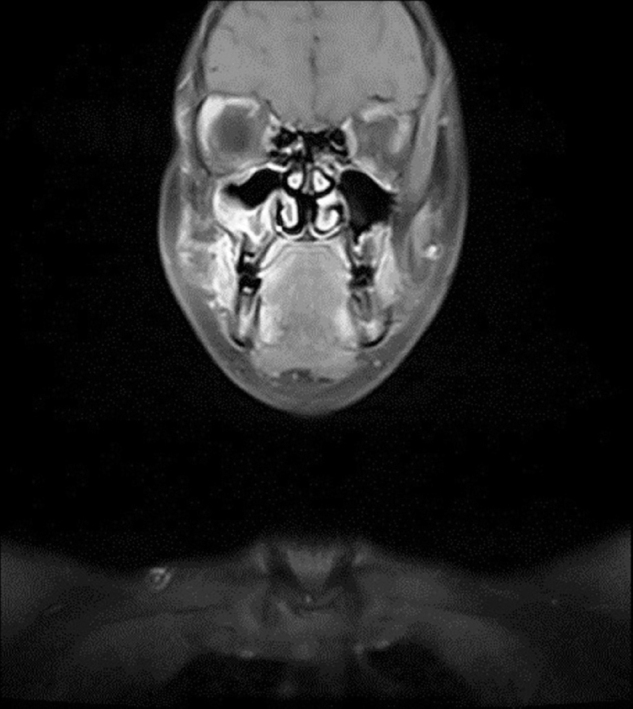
A coronal MRI of the sinuses; mucosal thickening appears in the right maxillary sinus, after treatment.
